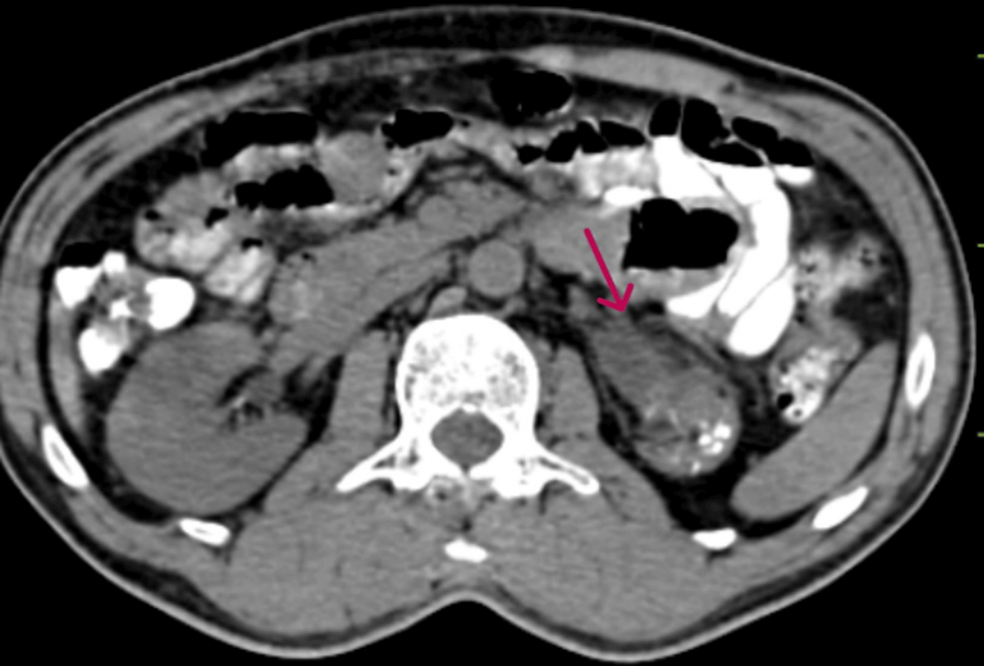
A contrast-enhanced CT of the abdomen and pelvis of the patient shows a small, shrunken, and atrophic left kidney with marked thinning of the cortex and distorted parenchyma. The extrarenal pelvis is seen on the left side.
